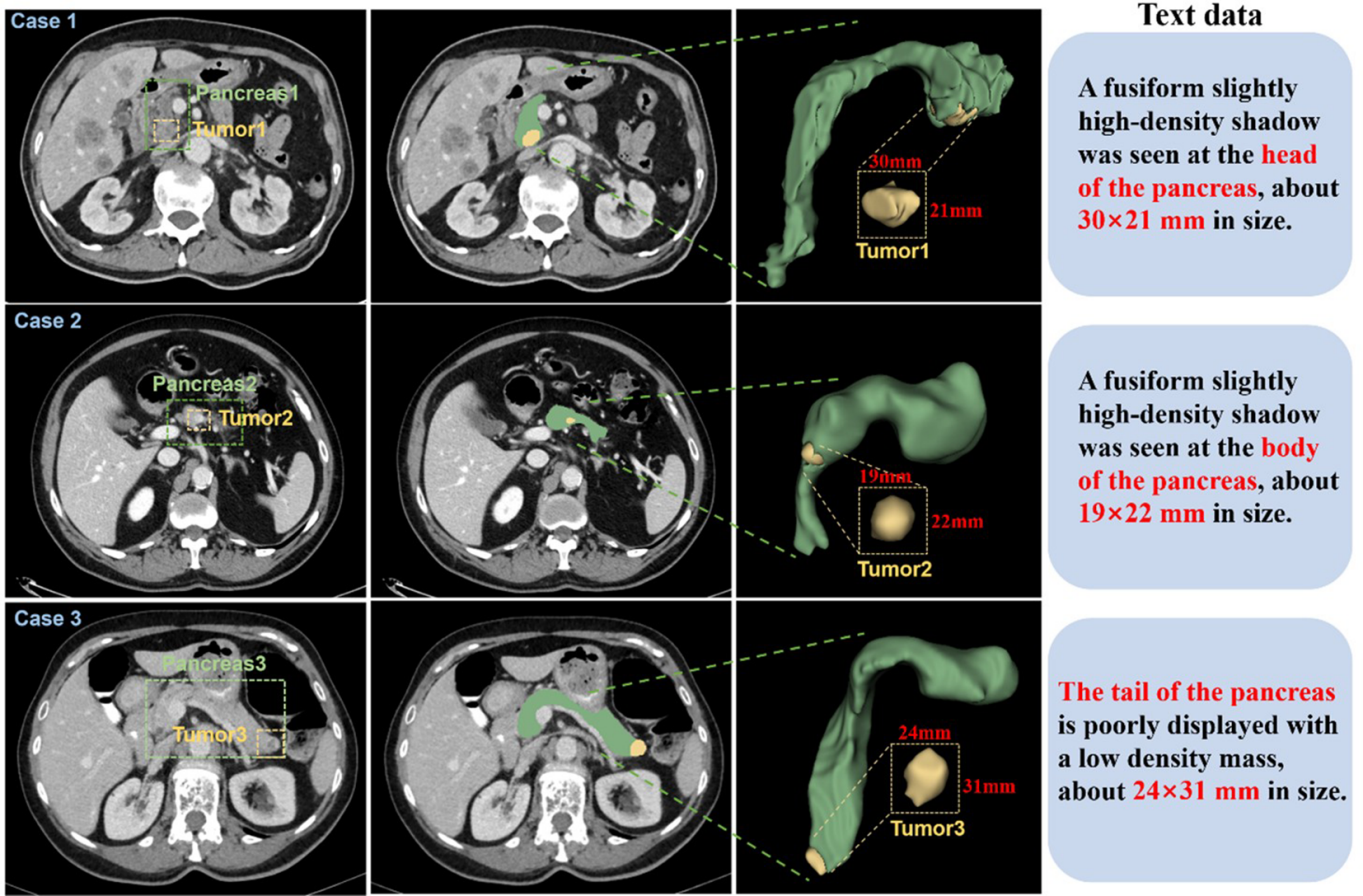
CT images of 3 patients were selected from the self-constructed dataset. Each case displays (left to right): three magnified axial slices, 3D reconstruction, and associated clinical annotations. Pancreatic anatomy is demarcated by green dashed boundaries, with yellow dashed contours highlighting tumor regions.
